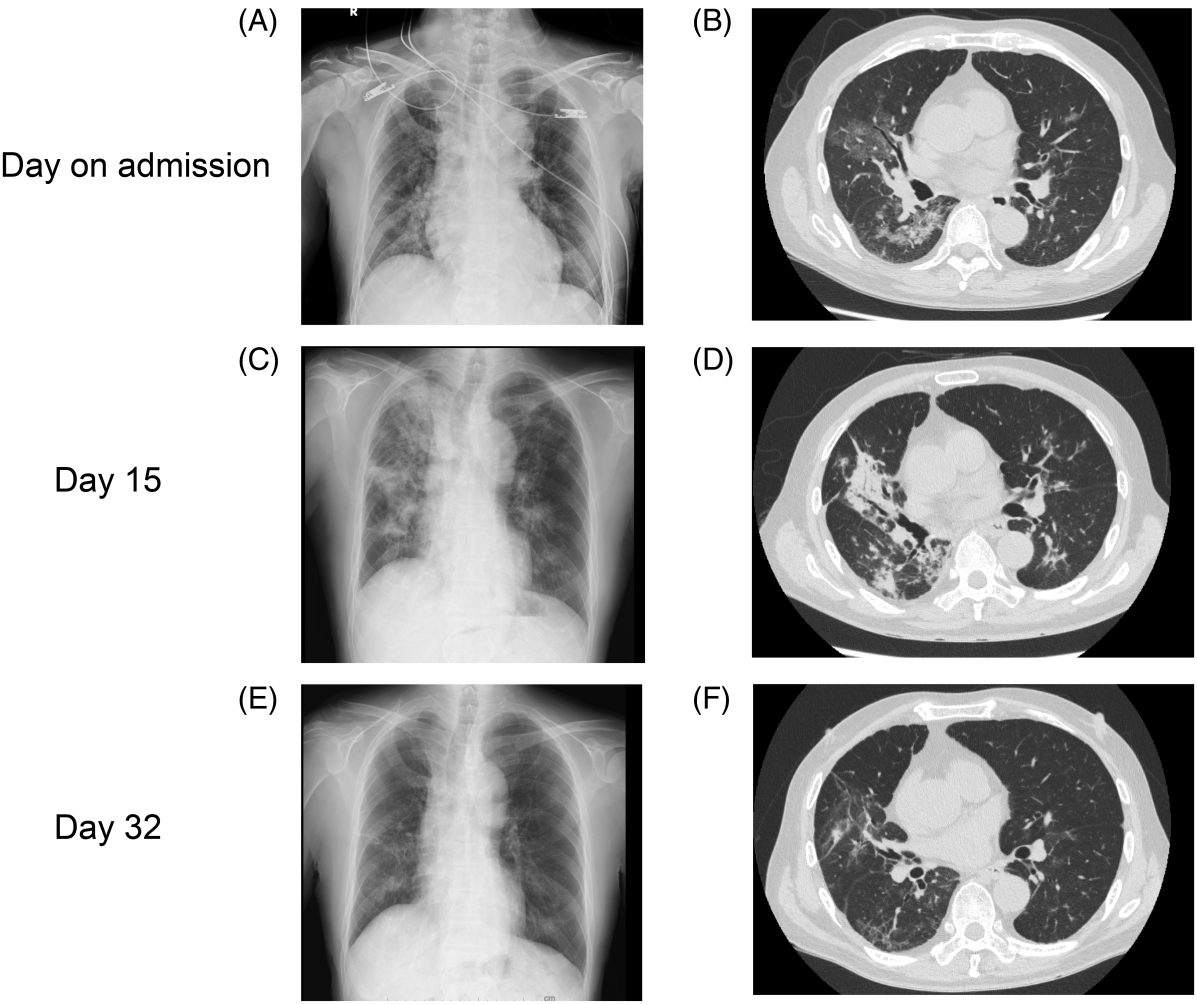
Chest radiograph and computed tomography (CT). Chest radiograph and CT scan showed bilateral ground‐glass attenuation on admission (A, B). Chest radiograph showing shadow exacerbation predominantly in the right lung (C) and CT scan showing consolidation predominantly in the right lung (D) on day 15. Chest radiograph showing decreased shadow (E) and CT scan showing decreased consolidation in bilateral lung (F) on day 32.
